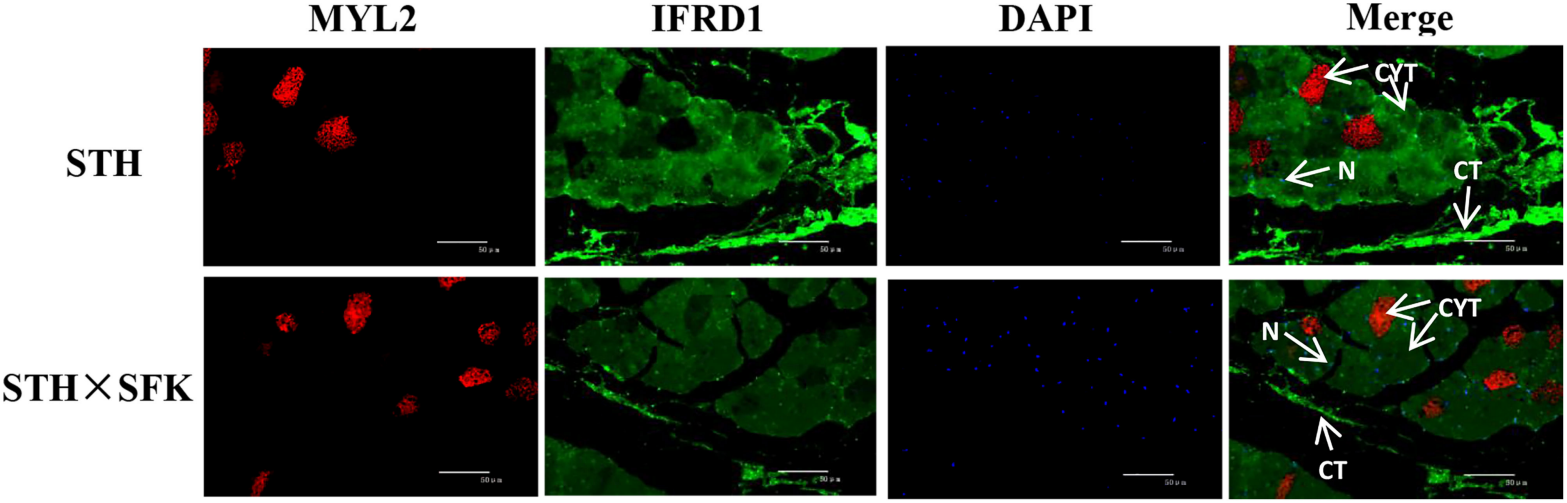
Expression location of MYL2 and IFRD1 protein in STH and STH×SFK *longissimus dorsi* muscle (IF×400). CYT, Cytoplasm; N, Nucleus; CT, Connective tissue.
